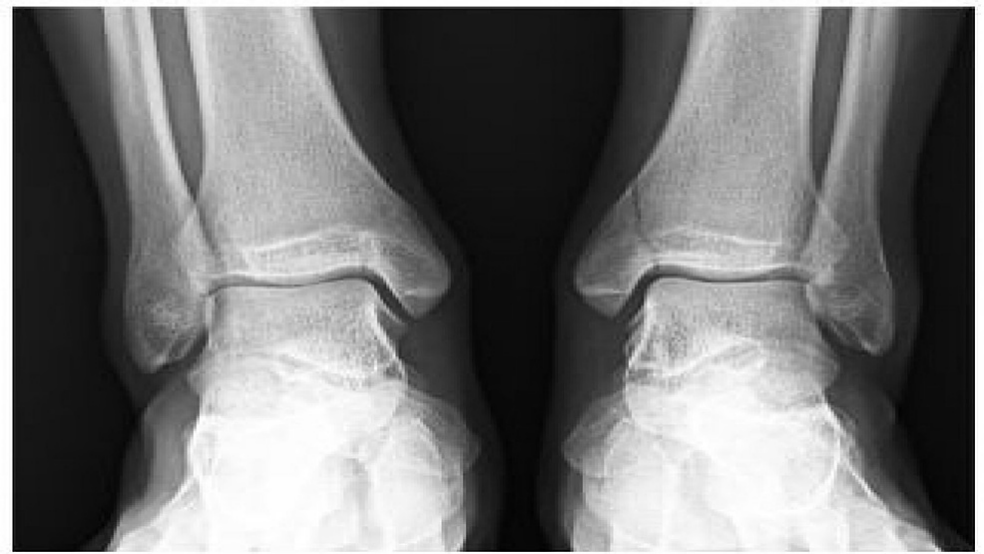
Pre-operative bilateral AP ankle radiograph demonstrating complete non-displaced medial malleolus stress fracture on the left, and incomplete medial malleolus stress fracture on the right. AP, anteroposterior.
